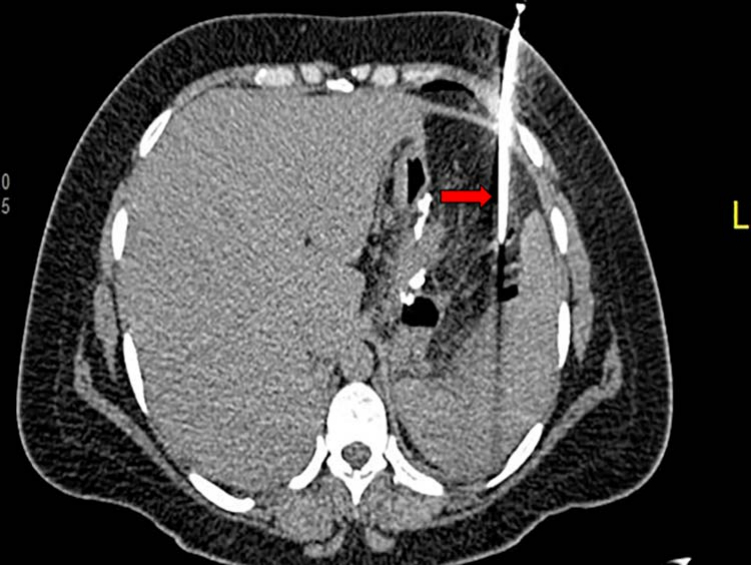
CT guided drain of intra-abdominal collection.
